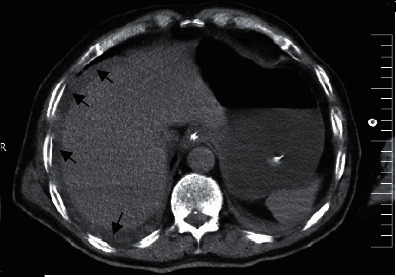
Male patient's abdominal CT (arrows: ascites).
